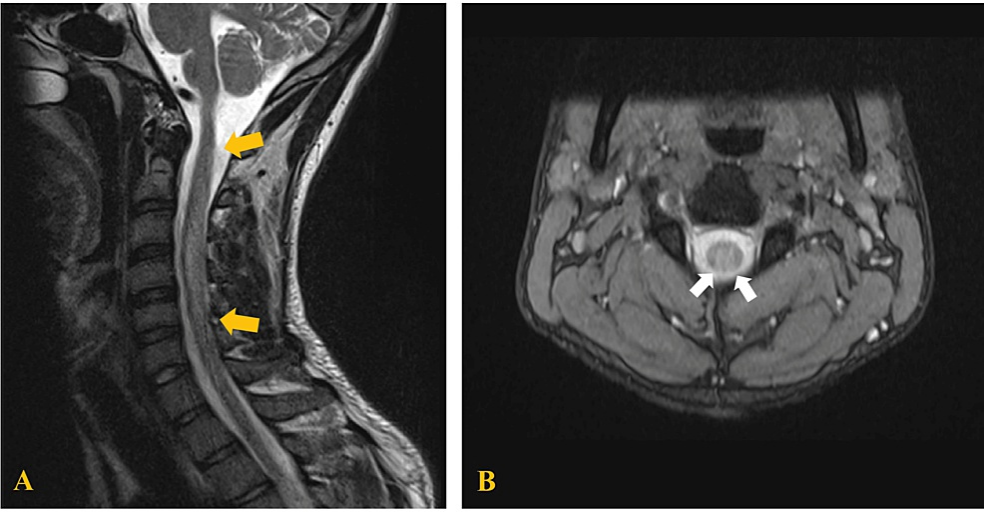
Magnetic resonance imaging (MRI) of the cervical spine of patient 1. (A) T2-weighted sagittal image of the cervical spine, highlighting the hyperintensity of dorsal columns involving C1 through C5 segments (yellow arrows). (B) T2-weighted axial image with the hyperintensity of dorsal columns (white arrow).
